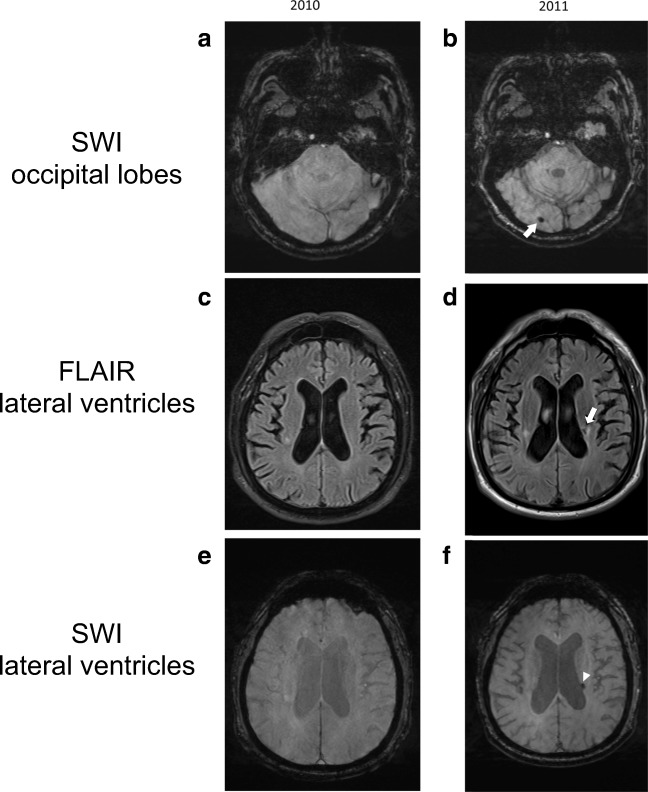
Representative magnetic resonance images showing progression of brain pathology in a chronic hemodialysis patient. The patient had imaging done in 2010 (images A, C, and E) and in 2011 (images B, D, and F). (A, B) SWI (susceptibility-weighted imaging) at the level of the occipital lobes demonstrate an interval microbleed within the right occipital lobe (white arrow). (C, D) FLAIR (fluid attenuated inversion recovery) images at the level of the lateral ventricles demonstrate an interval lacunar infarction of the left periventricular white matter (white arrow). (E, F) SWI images through the lateral ventricles demonstrate blood products associated with this lesion, compatible with a hemorrhagic infarction or microinfarction
